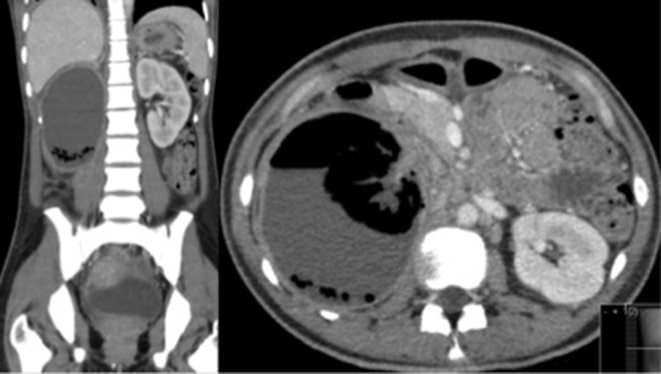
CT image of abdominal windows demonstrating persistent emphysematous change with new 11 × 9 × 12  cm air-fluid collection around the right kidney.
